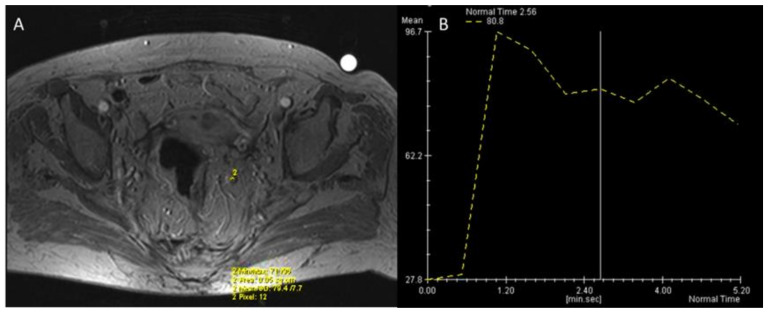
Man 72 y.o. with rectal cancer (same patient as in Figure 3 and Figure 5). DCE- MRI (A) nodal assessment with Intensity/Time curve evaluation (B).
